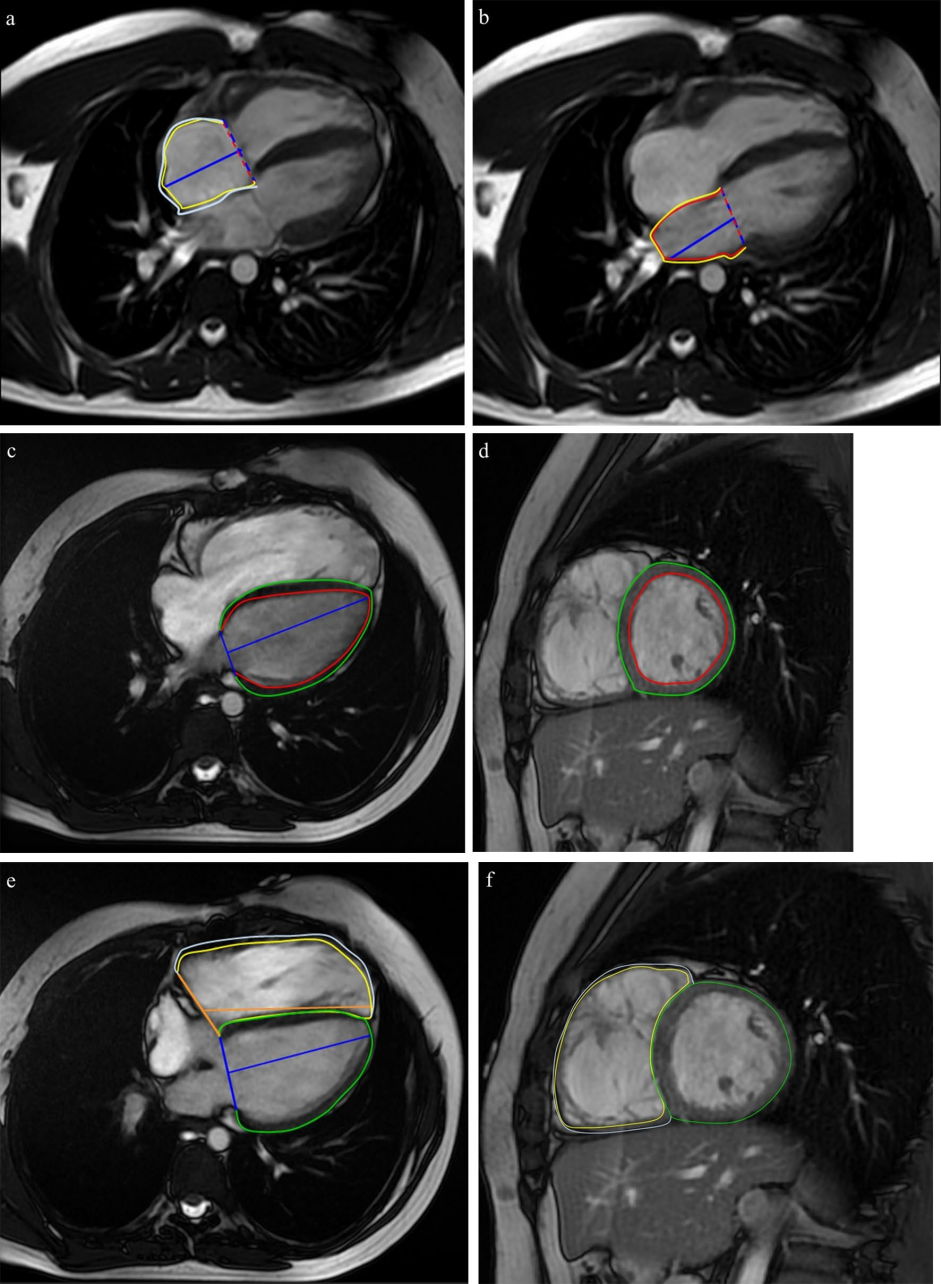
Representative feature-tracking strain cardiac MRI in a16-year-old male with repaired tetralogy of Fallot (a–f). Atrial strain measurement in four-chamber cine images for right (a) and left atria (b). Strain measurment of left (c, d) and right (e, f) ventricles in four-chamber and short axis cine steady-state free precession images to produce the circumferential longitudinal, and radial strain curves
